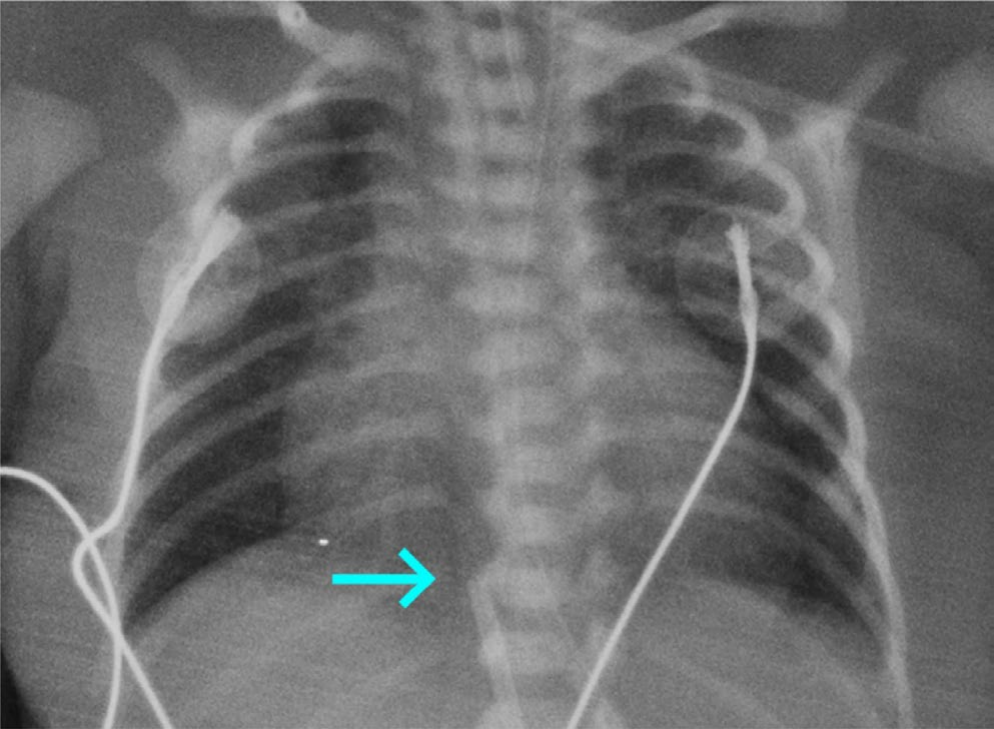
Chest radiography shows the acceptable catheter tip position at the level 10th thoracic vertebrae
